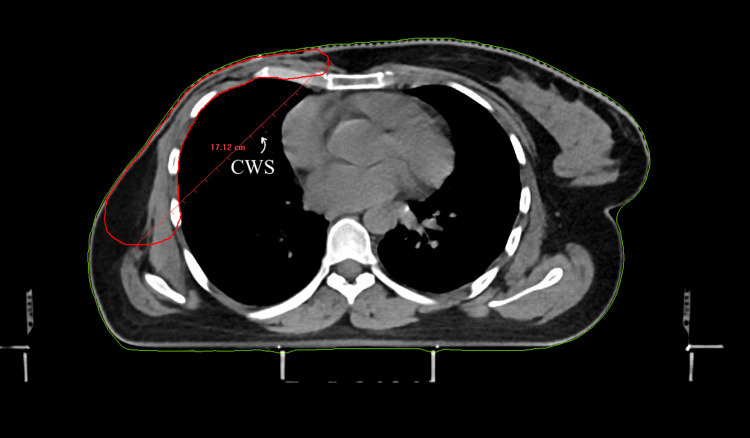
Axial planning CT image demonstrating the method used to measure chest wall separation (CWS). The contoured chest wall target volume is outlined (red). CWS is defined as the tangential distance between the anterior and posterior extents of the contoured chest wall on an axial slice, measured along the beam direction. The measurement shown represents one of three central slices used for averaging to obtain the final CWS value for each patient.
